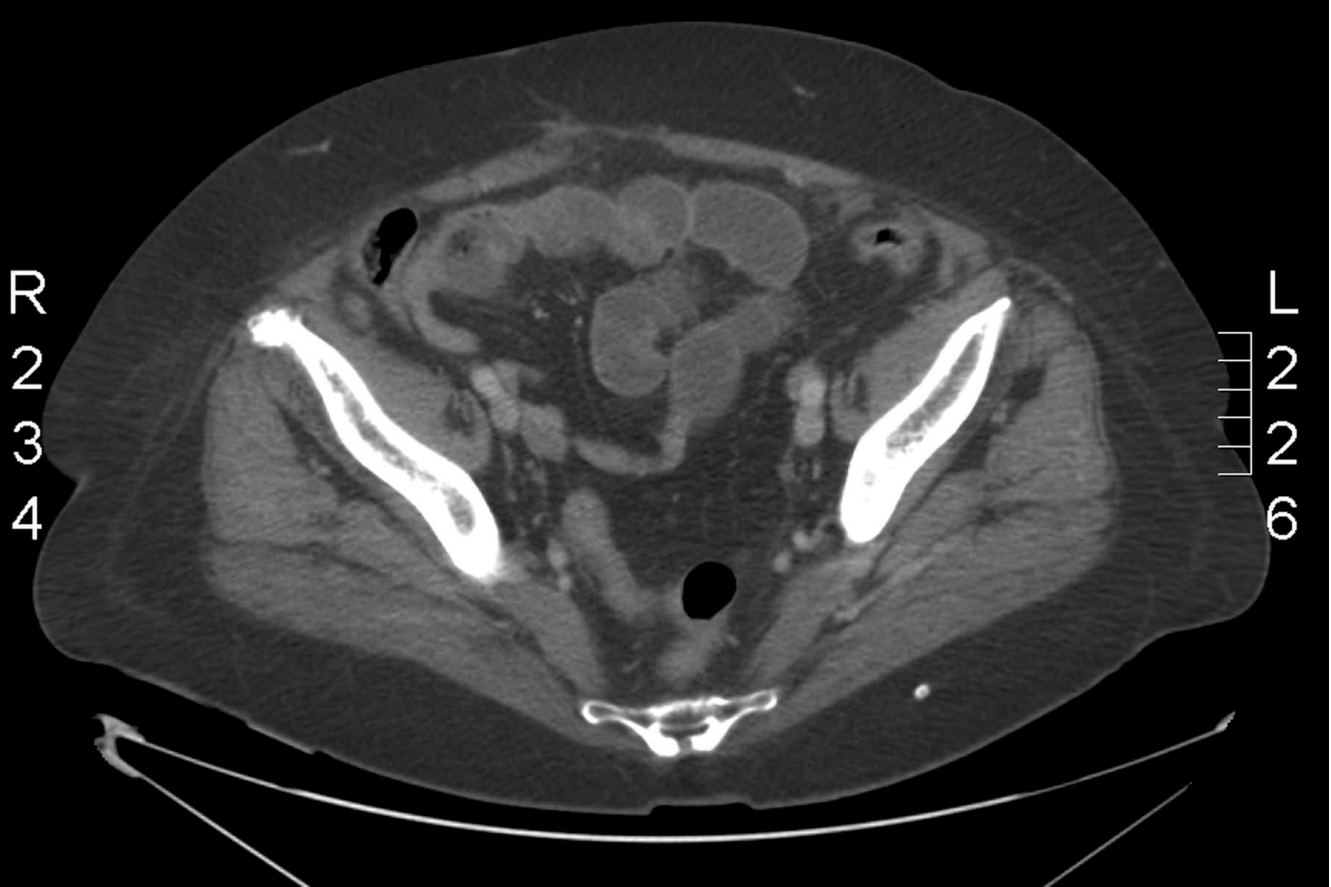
CT scan demonstrating mechanical small bowel obstruction.
